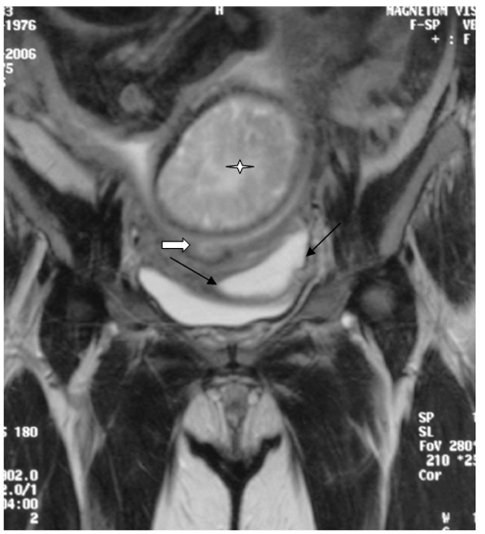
Coronal T2-weighted image shows the cyst (black arrows) lying lateral to the cervical os (block arrow). The fetal brain (asterisk) is seen within the uterus.
